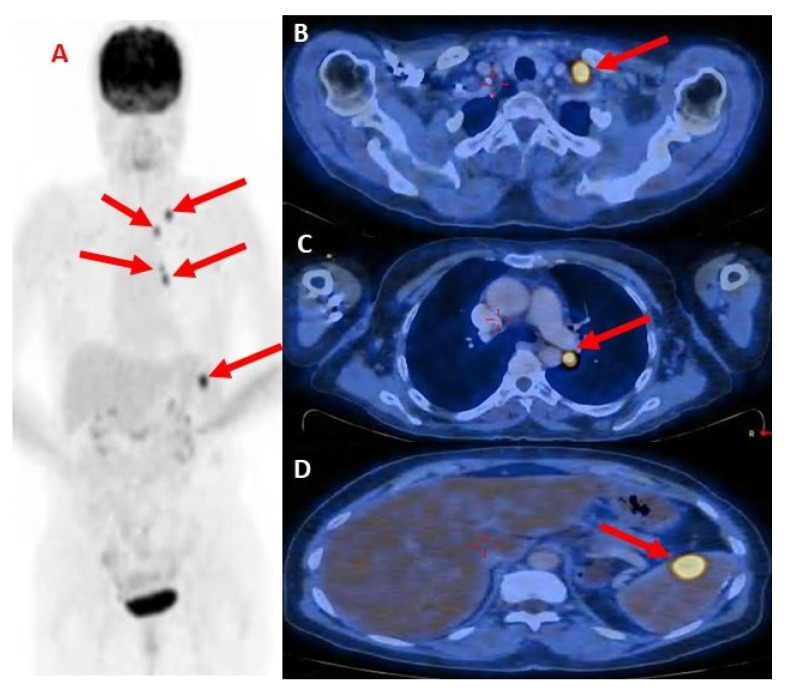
(A) PET-CT scan showing the spread of metastatic disease; (B) showing hyper-metabolic uptake in the LUL mass; (C) Hypermetabolic uptake and enlargement of the lymph nodes in the left supraclavicular area; and (D) hyper-metabolic uptake in the spleen. Red arrows indicate metastasis.
